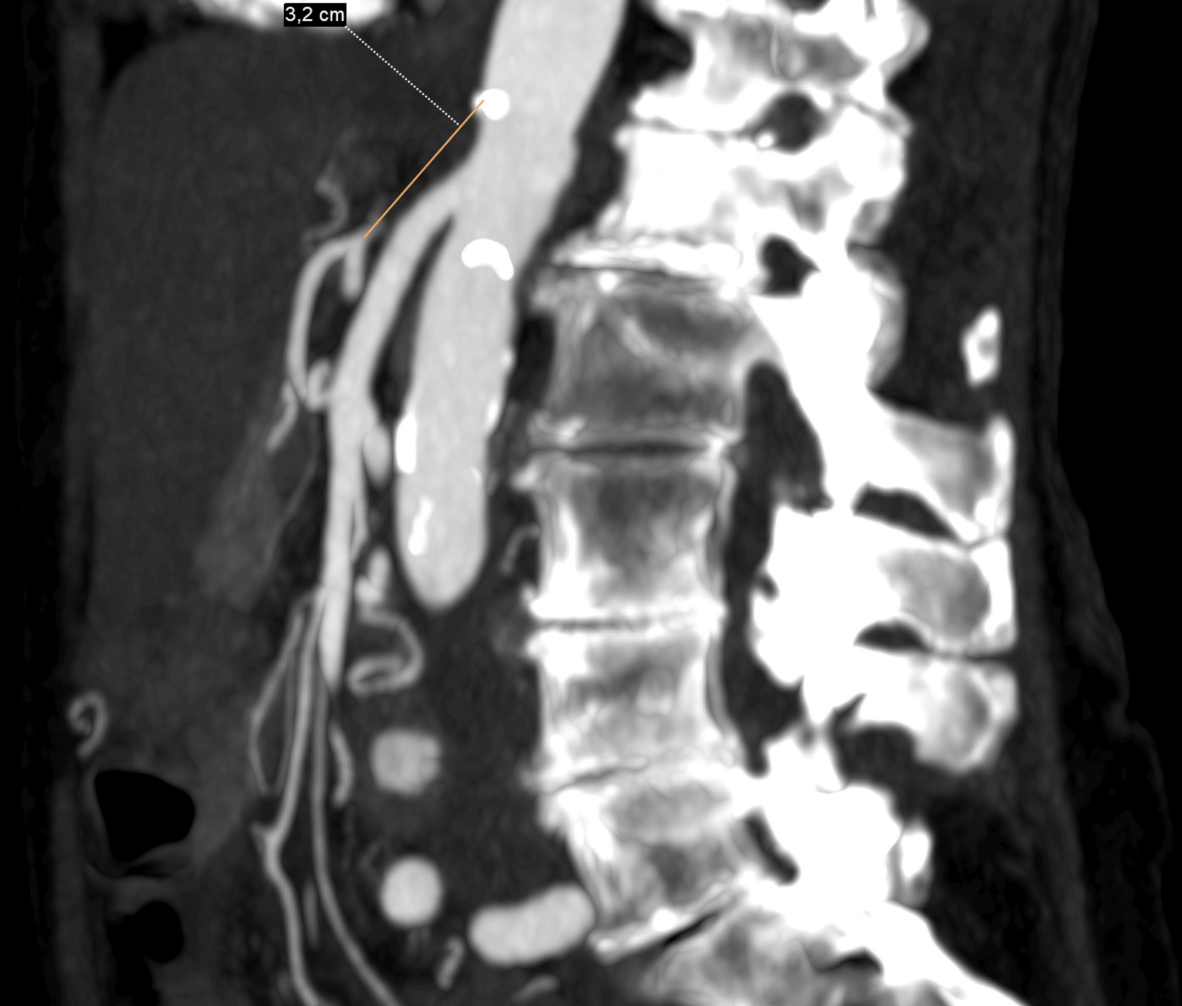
CT angiography sagittal maximum-intensity-projection (MIP) demonstrating a steep aortomesenteric angle and complete long-segment chronic occlusion of the celiac trunk without a residual ostium.
